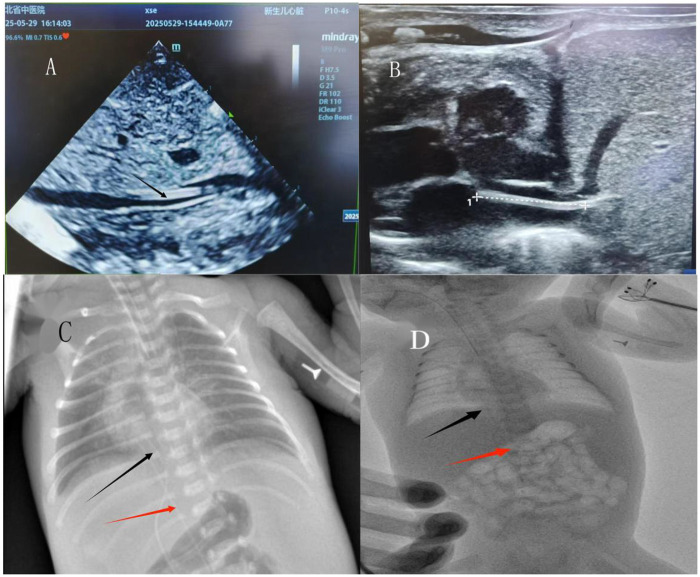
Preoperative imaging findings: (A, B) cardiac echocardiography. (C) Preoperative bedside x-ray (red arrow: the femoral venous PICC catheter, black arrow: the residual segment of the umbilical venous PICC catheter). (D) Preoperative fluoroscopic imaging (red arrow: the femoral venous PICC catheter, black arrow: the residual segment of the umbilical venous PICC catheter).
